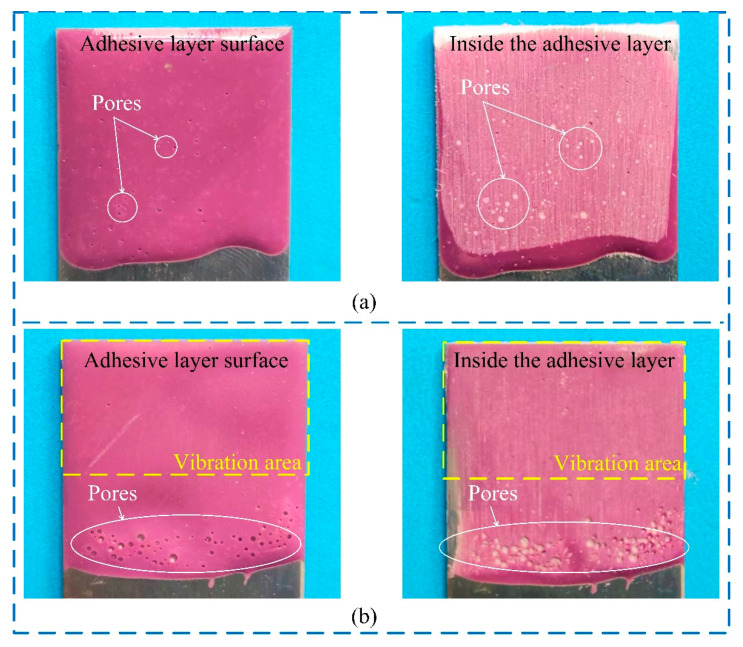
Pores in the cured adhesive (a) without and (b) with the ultrasonic vibration treatment.
